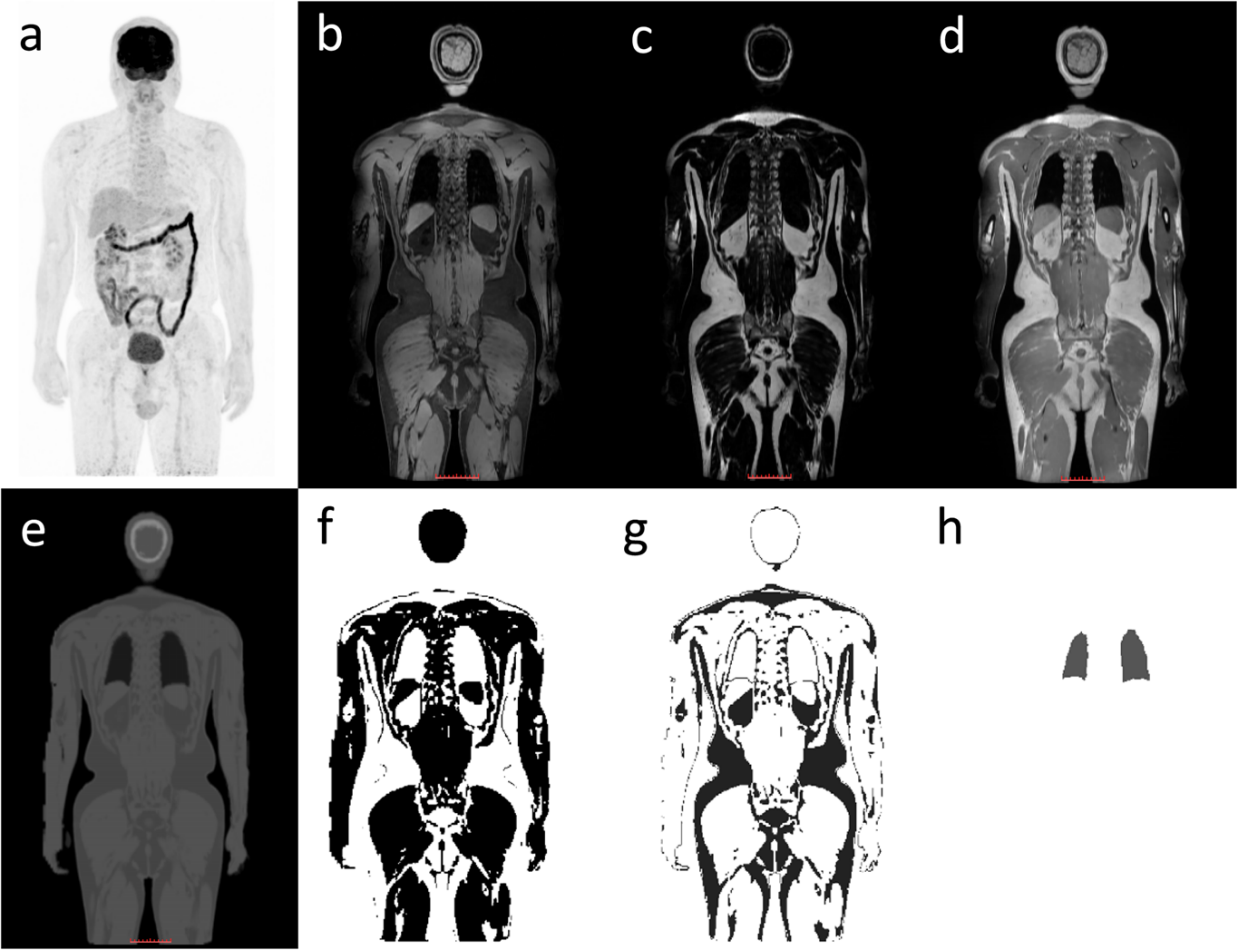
Representative images from a ^18^F-FDG PET/MR scan. a PET MIP, b water image, c fat image, d in-phase image, e MRAC map, f water segmented from MRAC, g fat segmented from MRAC, and h lung segmented from MRAC
